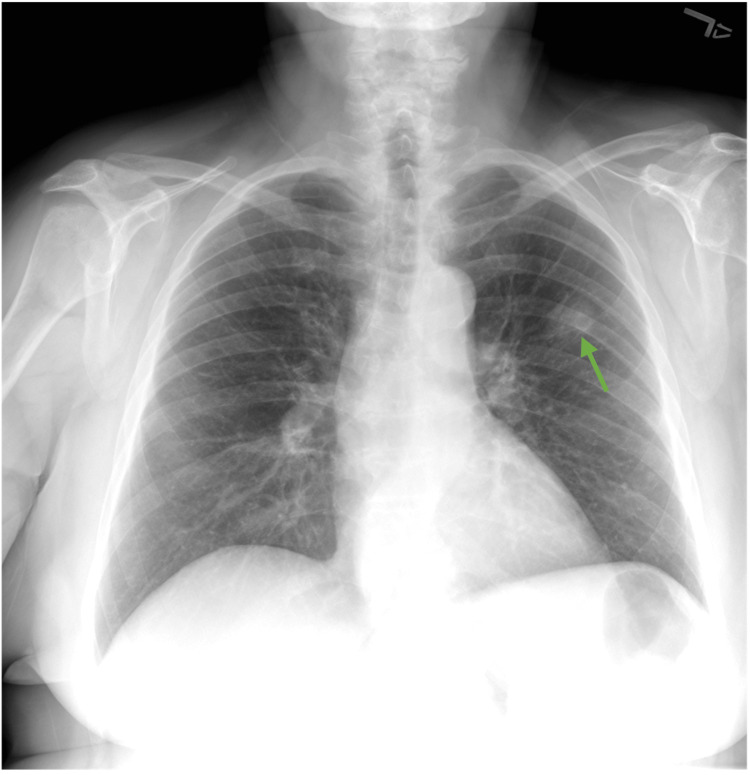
Initial PA chest radiograph revealing a 2 cm left upper lobe nodule (green arrow) PA: posteroanterior
